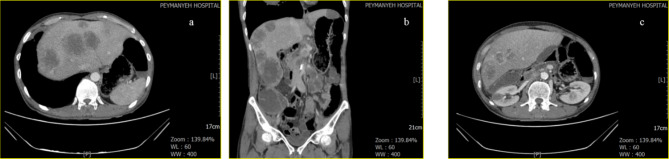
Abdominopelvic CT scan a, b and c) Axial, coronal and axial view depicting multiple lesions in liver and kidney
